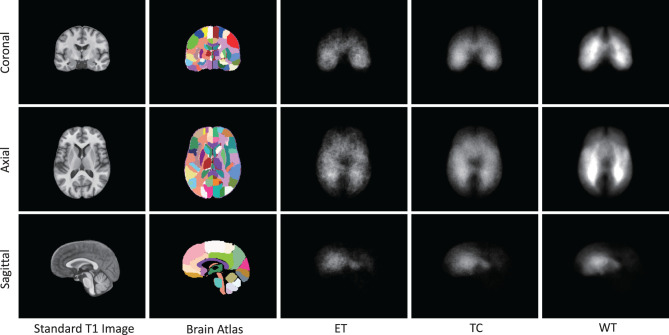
The occurrence frequency in SRI-24 standard space among 1,251 cases from BraTS21 dataset. The five columns represent the standard brain T1 MR image, brain atlas (enhanced parc116 plus) in SRI-24 Space, Enhanced Tumor (ET) occurrence, Tumor Core (TC) Tumor occurrence, and Whole Tumor (WT) occurrence, respectively.
